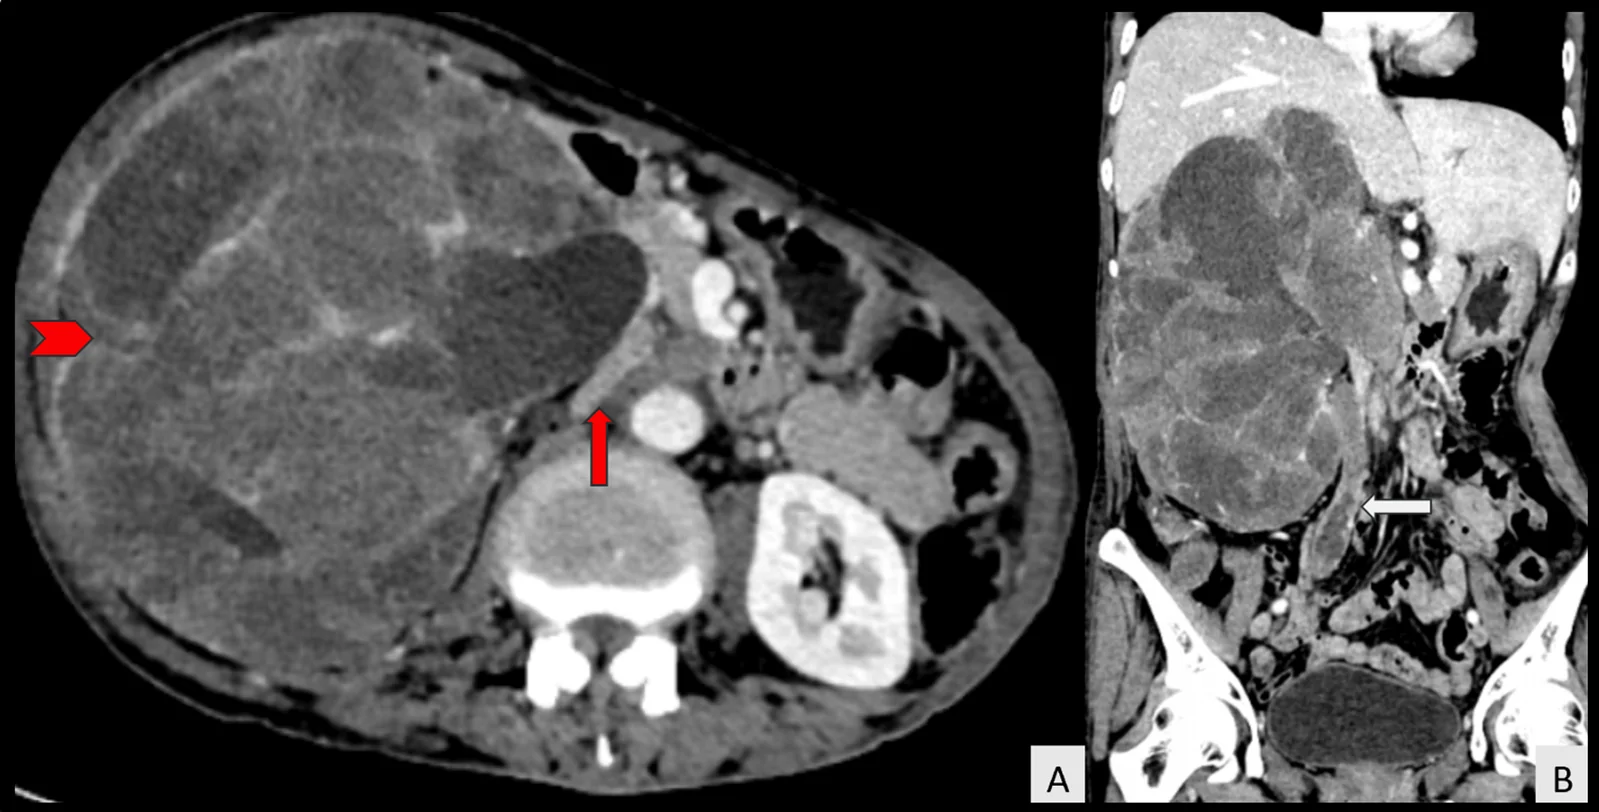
CECT of the right renal mass with collecting system involvement A: Axial corticomedullary CT urography image demonstrates a large heterogeneously enhancing right renal mass (red arrowhead) with multiple internal necrotic areas, causing marked compression of the IVC (red arrow) without evidence of vascular invasion. B: Coronal reconstructed corticomedullary phase of CT urography image demonstrates preservation of the reniform configuration of the kidney with contiguous involvement of the pelvicalyceal system and enhancing soft tissue extending along the entire right ureter up to the vesicoureteric junction (white arrow), closely mimicking transitional cell/urothelial carcinoma. IVC: Inferior vena cava
